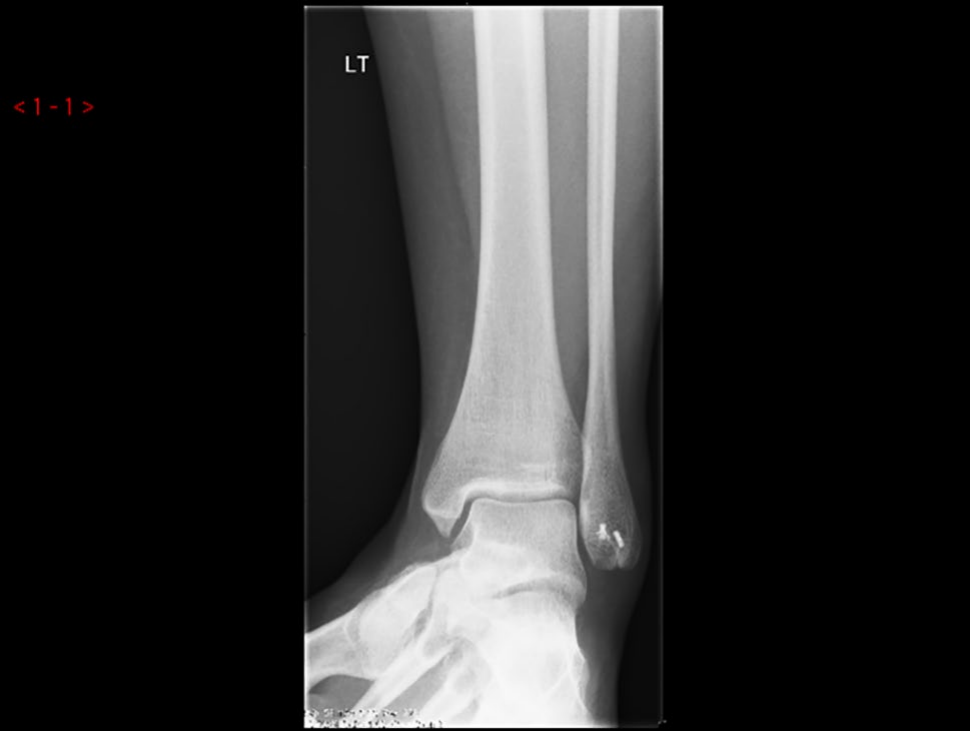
Post-operative X-ray: resection of os subfibulare and repair of the ATFL with anchors
